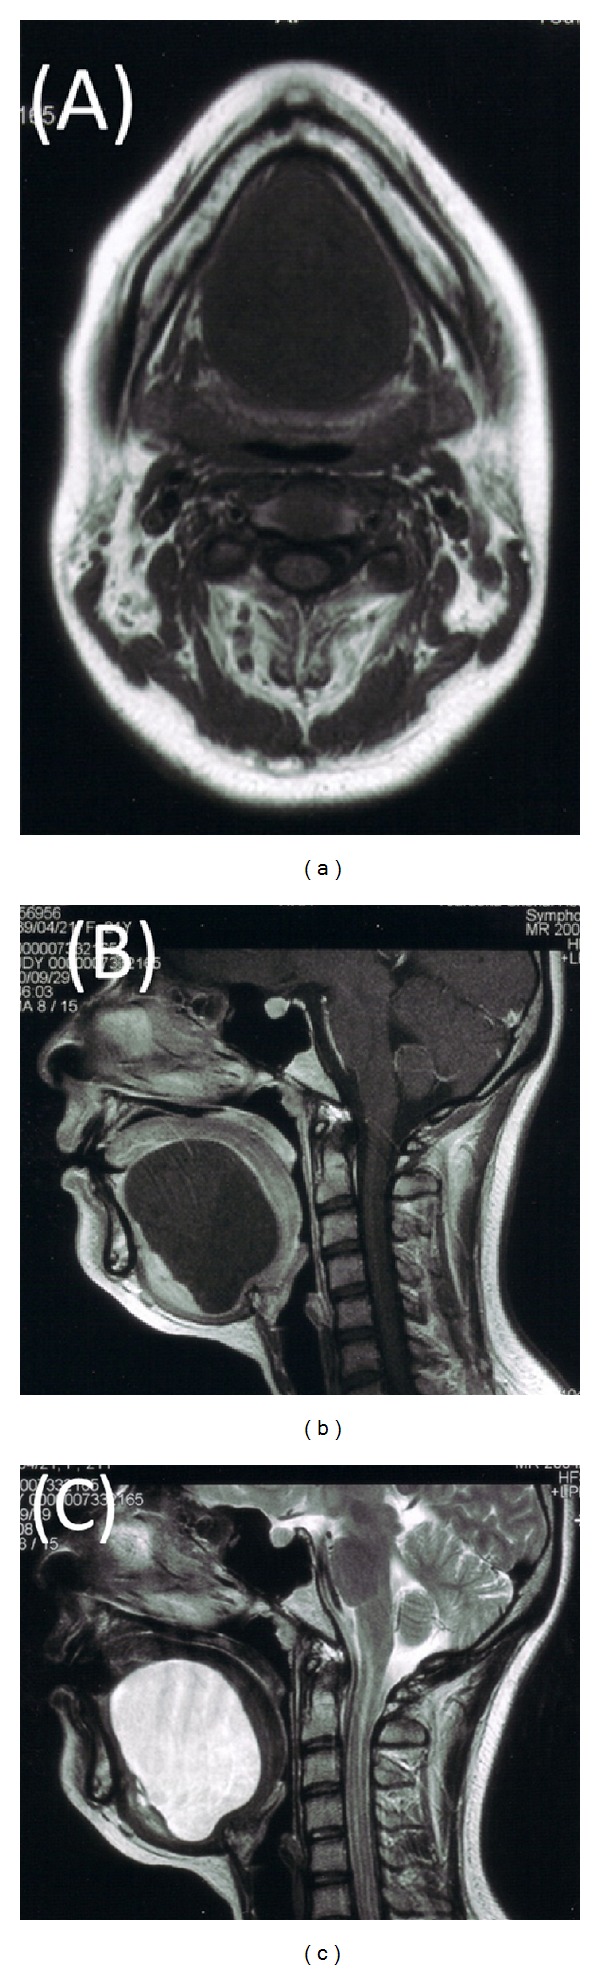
(a) Axial T1-weighted magnetic resonance imaging (MRI) shows a sharply circumscribed cystic mass. (b) Sagittal-T1 weighted MRI shows an 55 × 65-mm well-circumscribed cystic mass extending from the sublingual area to the mylohyoid muscle. (c) Sagittal T2-weighted MRI shows an high-intensity mass extending inferiomedially to the genioglossus muscle and inferiorly to the mylohyoid muscle.
